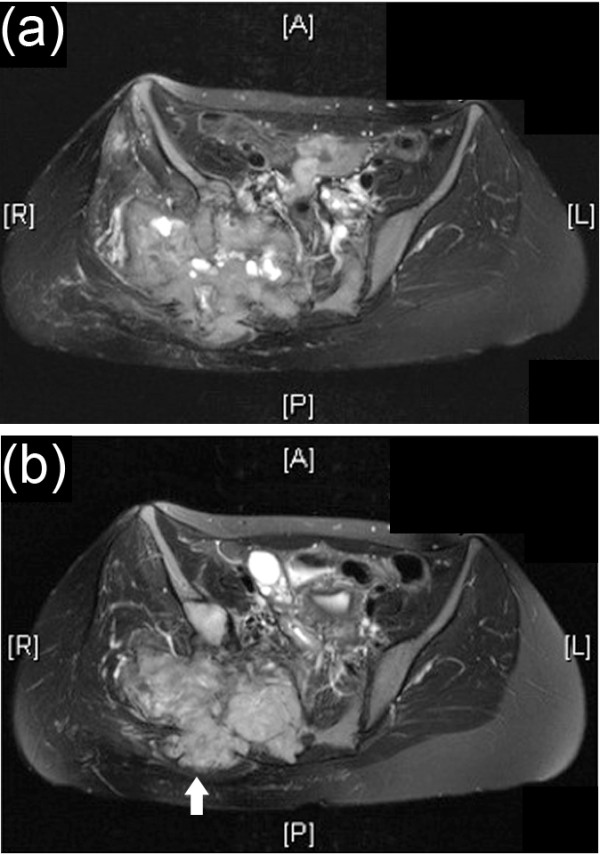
MRI of GCT pre and post zoledronic acid treatment. Axial T2- weighted (fat-suppressed) MRI of (a) pre and (b) 5 year post-zoledronic acid treatment of Case 12, an enlarging sacral/pelvic GCTB which shows stabilisation of tumour size. Following treatment there is some evidence of infilling of the lytic tumour (arrowed) following zoledronic acid treatment.
